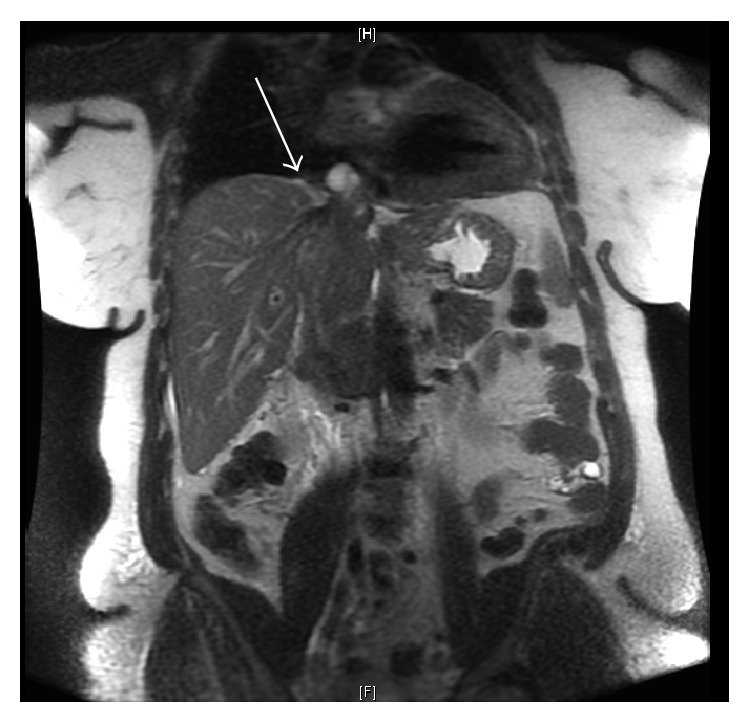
T1 MRI with sagittal view showing the tumor in the IVC with right atrial clot at the tip of the tumor (arrow).
